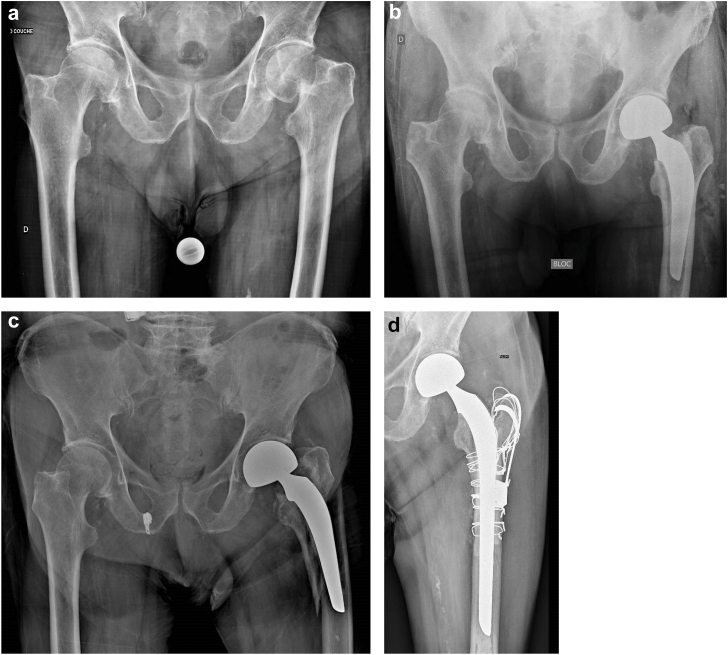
(A) Preoperative anteroposterior (AP) view of the pelvis in an 84-year-old male with left FNF with Dorr C femoral anatomy. (B) Immediate postoperative AP radiograph stem. (C) AP radiograph at 2 months postoperatively showing a PFF type B2 Vancouver following a fall. (D) AP radiograph performed in the follow-up 2 months after revision of the femoral component with a long cemented CK stem. FNF, femoral neck fracture.
